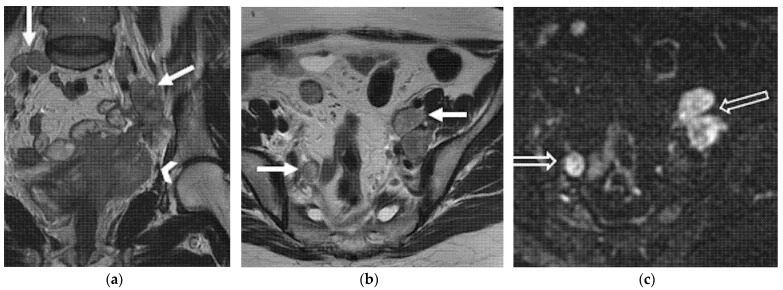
A 55-year-old patient who underwent radical hysterectomy with BSO and chemoradiation therapy for cervical cancer. (a) Coronal and (b) axial FSE T2-weighted images show multiple pelvic bilateral lymphadenopathies, with heterogenous signal intensity (arrows). Note the recurrent lesion in the vaginal cuff extended to the left parametrium (arrowhead in (a)). (c) The lymphadenopathies demonstrate restriction on diffusion-weighted image (open arrows).
